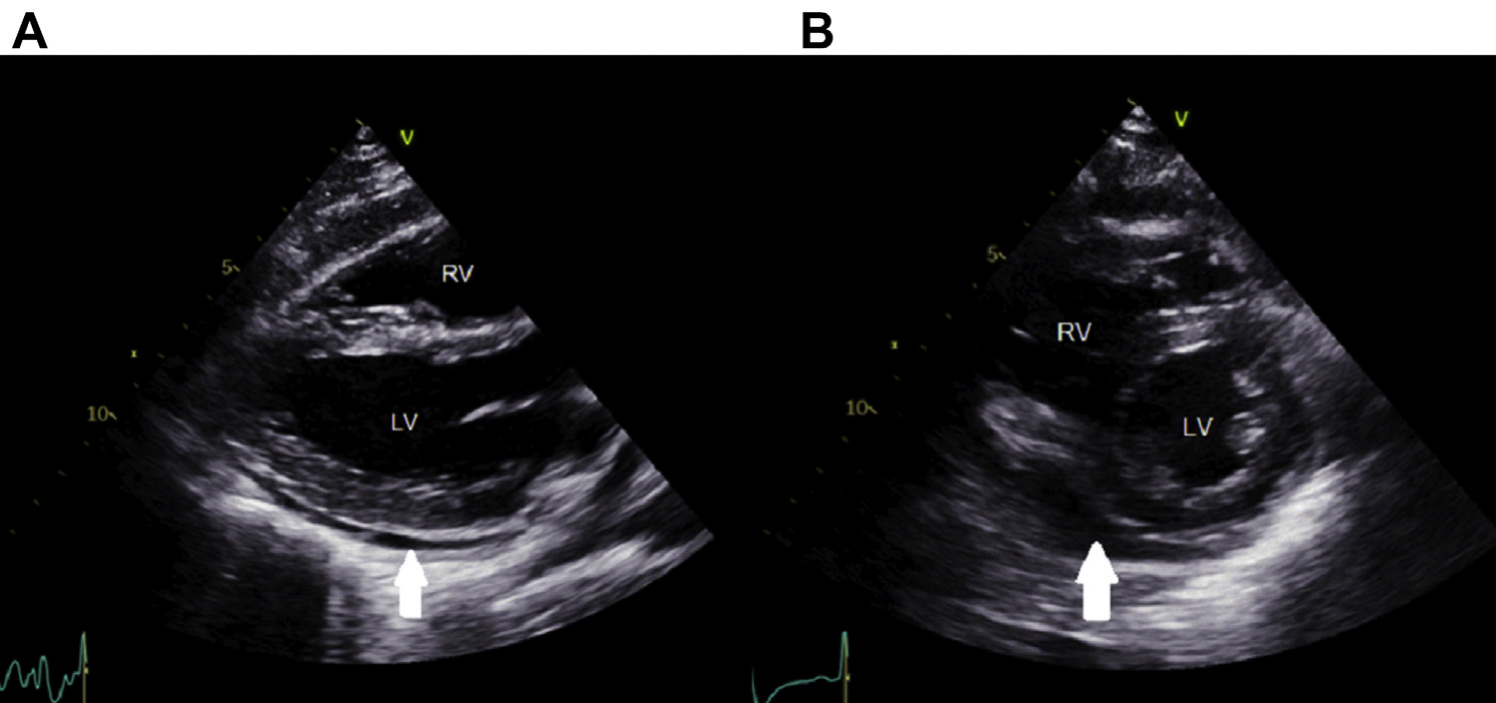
Spontaneous Improvement on Preprocedural Echocardiography (A) Parasternal long-axis and (B) parasternal short-axis views repeated 45 minutes after the previous echocardiogram. Spontaneous and significant improvement in the size of the pericardial effusion was noted (arrows). Abbreviations as in Figure 1.
